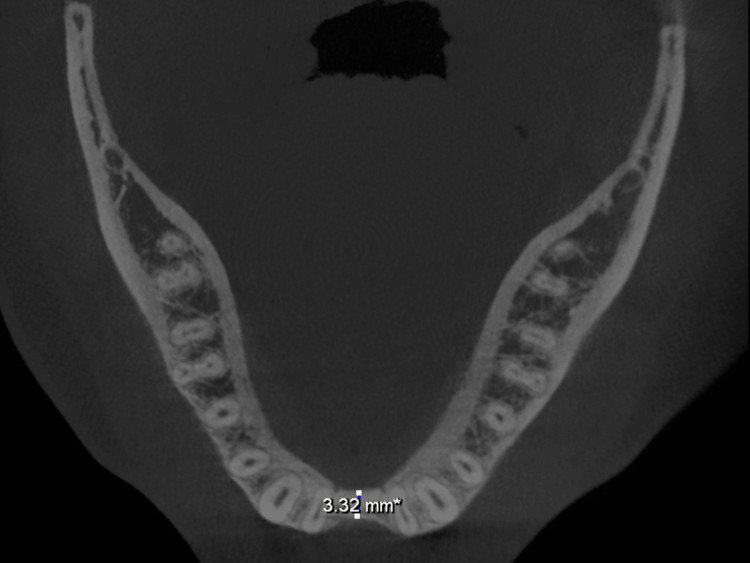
CBCT (axial view) indicating a preoperative mandibular ridge width of 3.32 mm. CBCT, cone-beam computed tomography
